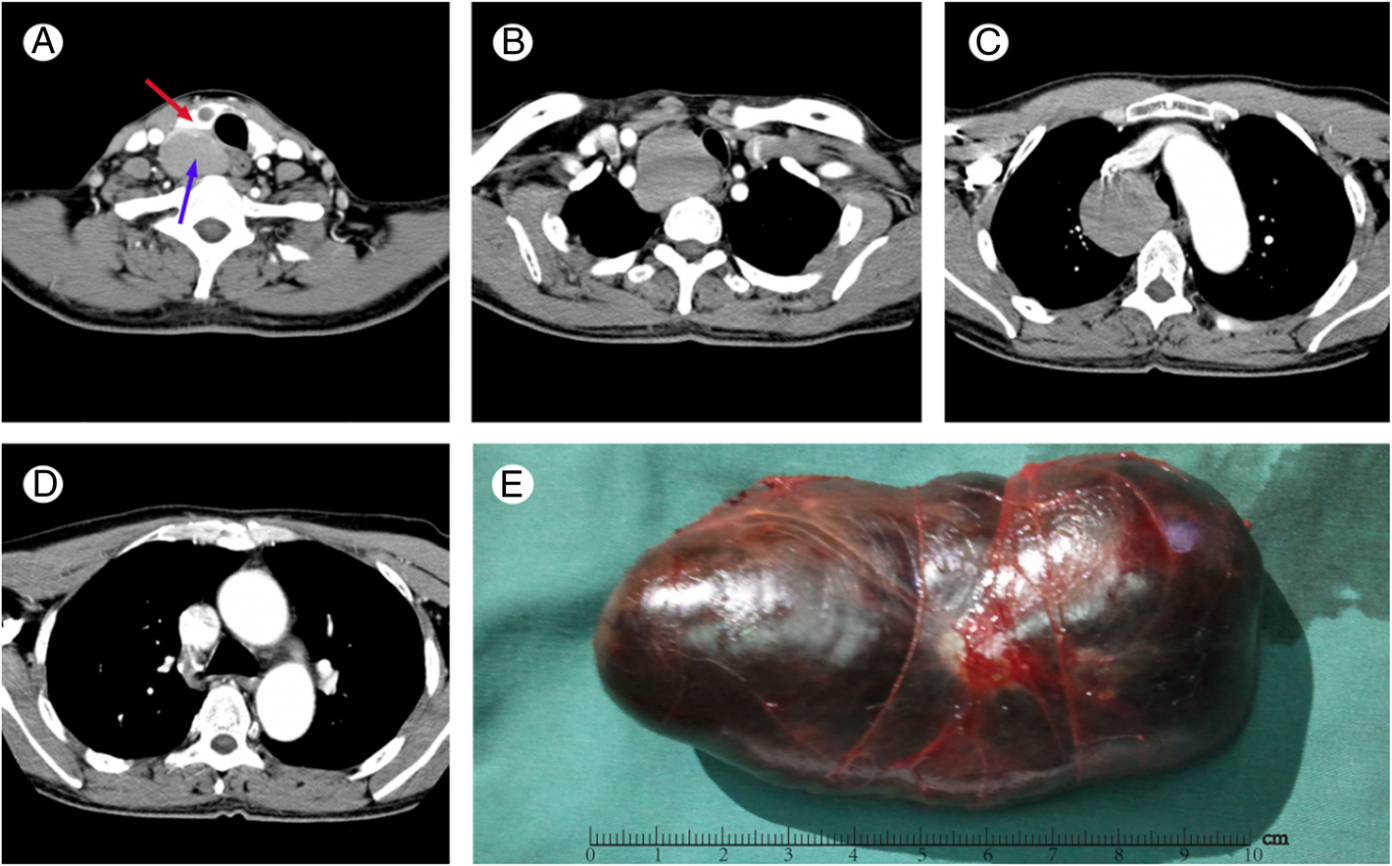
CT scanning and complete excision of giant thyroid goiter in posterior mediastinum. (A) Enhanced CT scanning reveals the right thyroid lobe (in red arrow) with a small cyst and a giant goiter (in blue arrow) in low density is on the back of the right lobe. (B) CT clavicle cross section reveals the giant goiter was located in the posterior mediastinum, compressing the trachea and esophagus. (C) CT of the chest reveals the goiter is well beyond the aortic arch and compressing the superior vena cava. (D) CT of the chest reveals the lower edge of the goiter reachs the carina of trachea. (E) The tumor is in a complete capsule with large tension, 10.0 × 9.0 × 9.0 cm in size.
